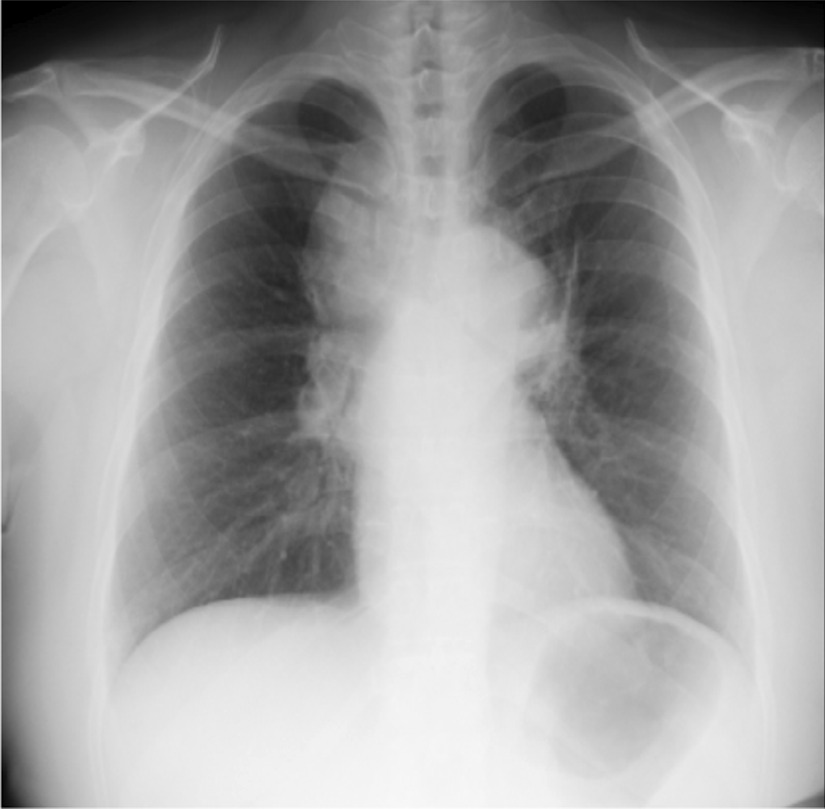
Chest X-ray showing an upper mediastinal widening
